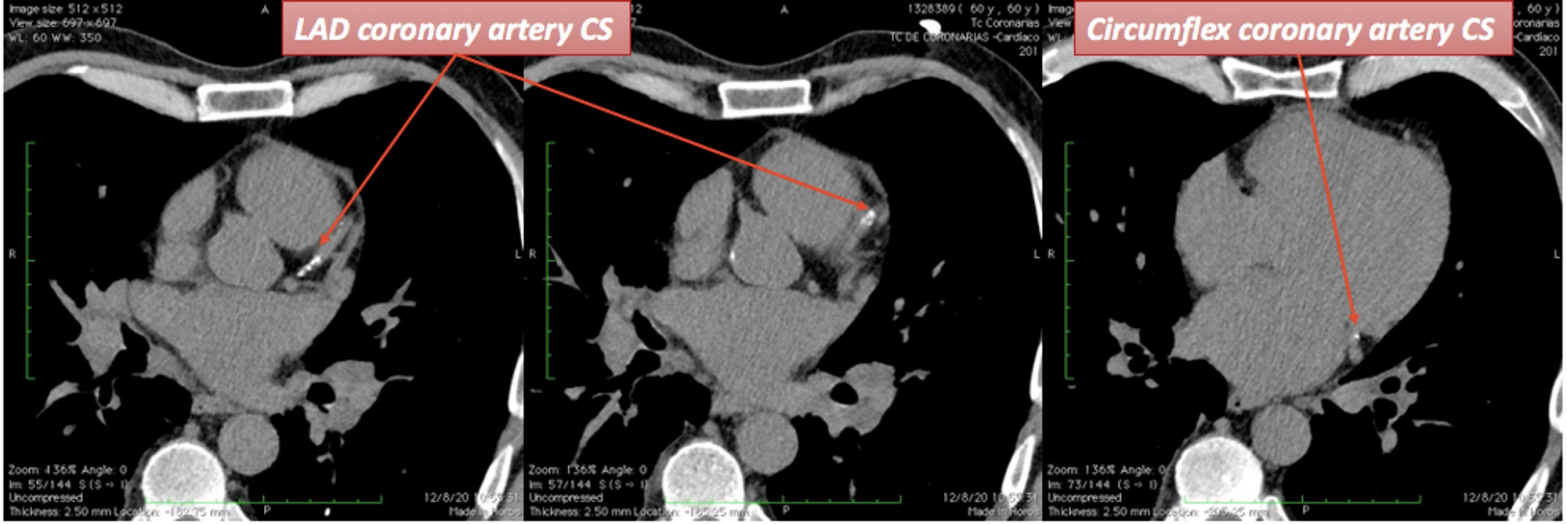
Non-contrast computed tomography coronary artery calcium score. LAD: Left anterior descending artery, CS: calcium score.
